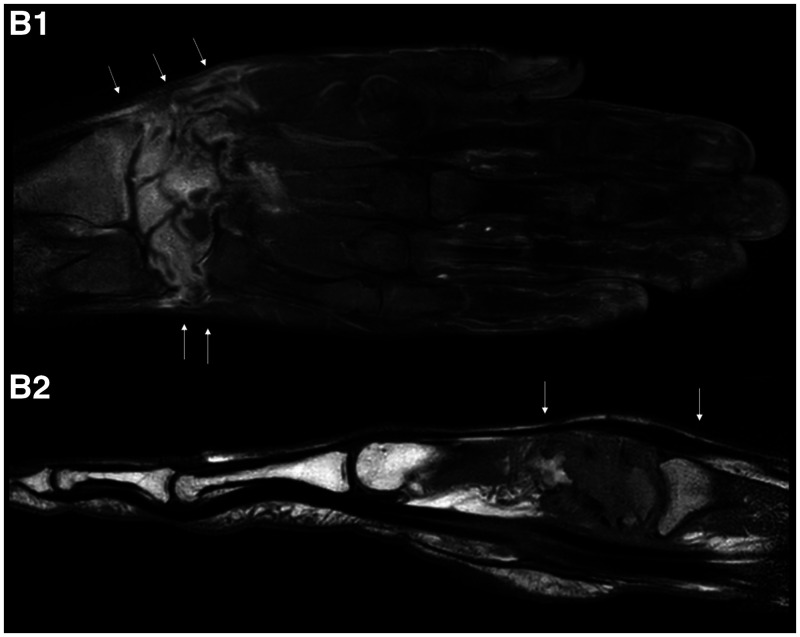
Contrast-enhanced magnetic resonance images of the hand showing synovitis, bone erosion, and pseudo-tumors extending up to the distal third of the forearm.
